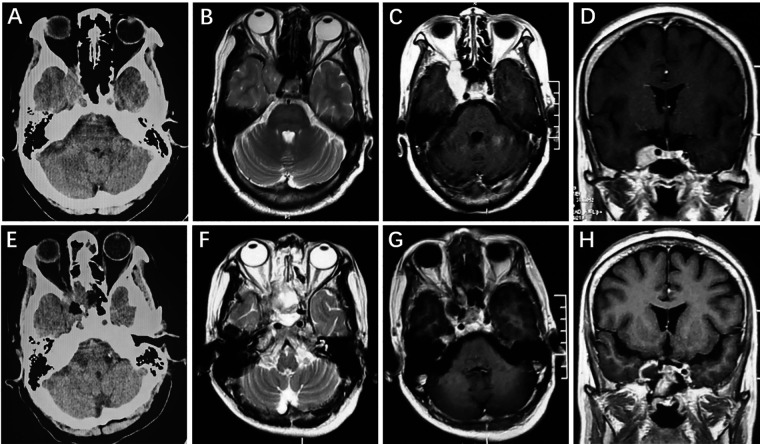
Right intracavernous HPC. Preoperative axial CT showed a slightly hyperintense lesion in the right cavernous sinus (A). Cranial axial MRI showed slight hyperintensity on T2-weighted imaging (B). After enhancement, the axial and coronal MRI showed homogeneous enhancement (C,D). Postoperative CT (E) and MRI (F–H) revealed that the tumor was completely removed.
